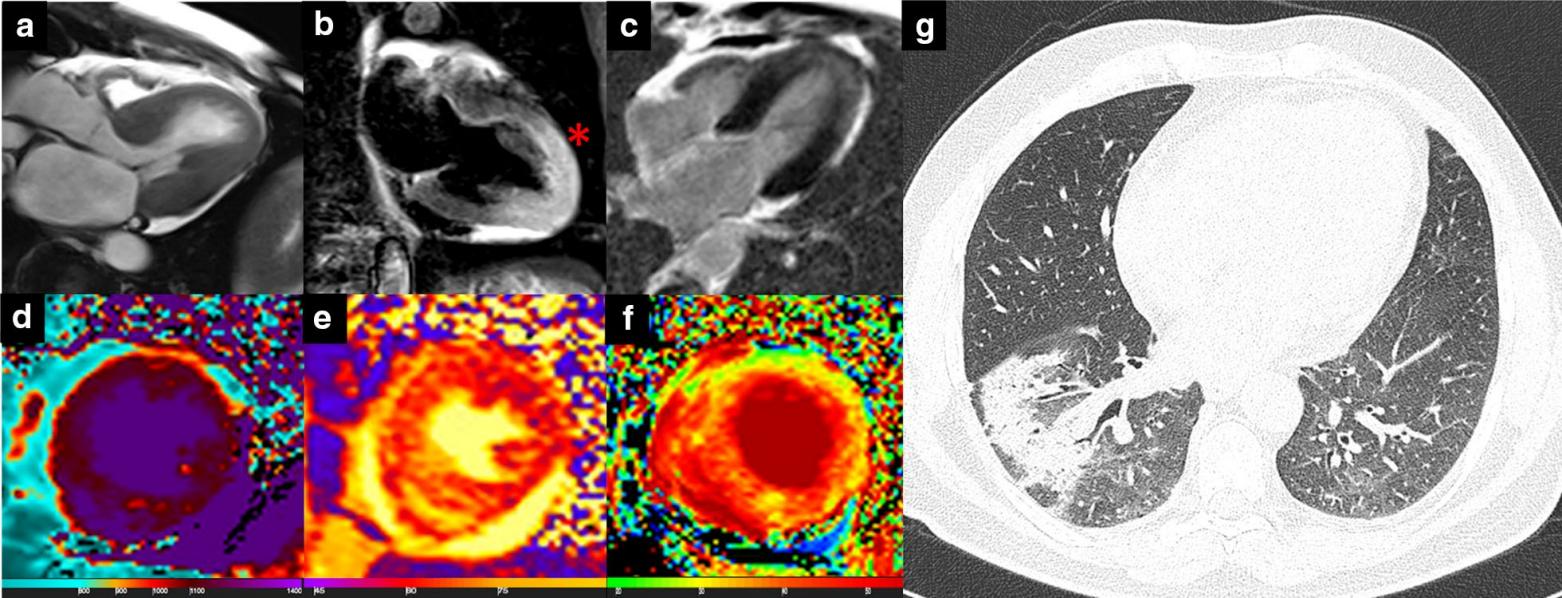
Takotsubo cardiomyopathy. COVID-19 female patient with chest pain and no CAD. Cine-SSFP showed a diffuse hypokinesia of mid and apical segments with «apical ballooning» sign (a). Diffuse edema of mid-ventricular and apical segments on 2-chambers STIR was found (b red asterisk). PSIR sequences evidenced no LGE (c). Native T1, T2 mapping and ECV fraction values were all increased (d–f). Chest CT showed a low-grade pulmonary involvement with peripheral interstitial thickening (g). *CAD* coronary artery disease; *SSFP* steady-state free precession; *STIR* short tau inversion recovery; *PSIR* phase sensitive inversion recovery; *LGE* late gadolinium enhancement; *ECV* extracellular volume; *CT* computed tomography
